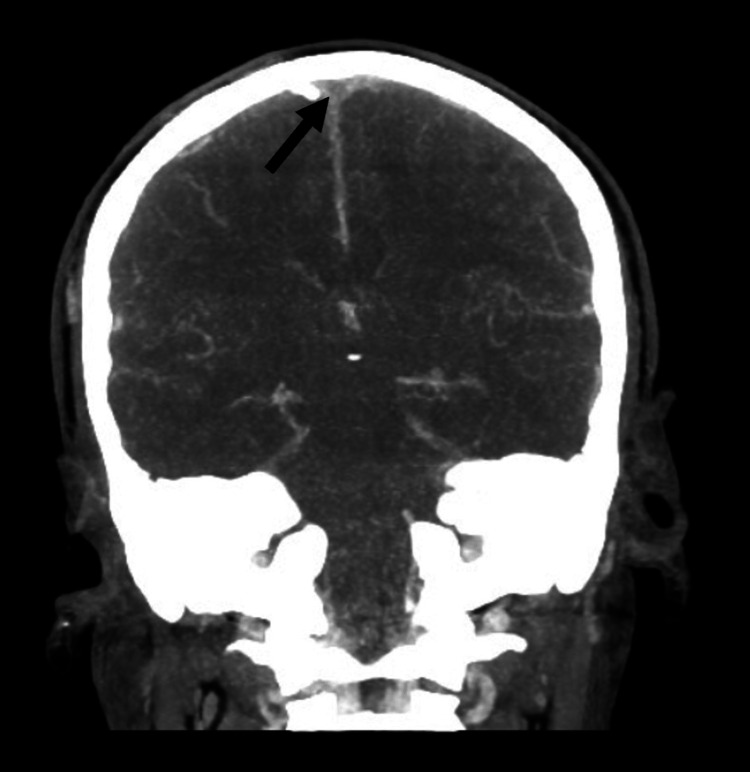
Brain CT venogram, coronal, showing non-opacified superior sagittal sinus giving the ‘empty delta sign’ (arrow)
